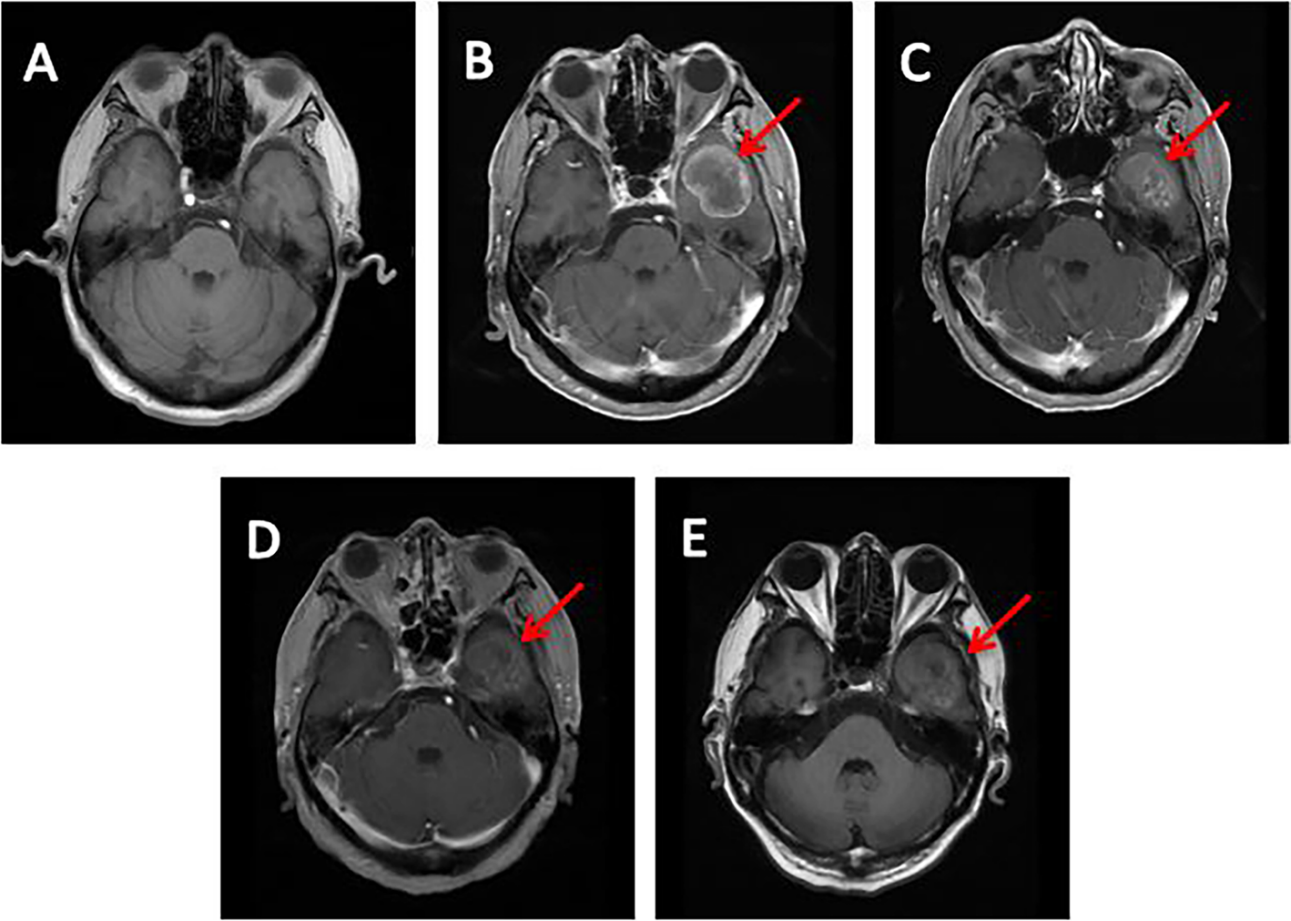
Brain magnetic resonance imaging (MRI) after treatment with afatinib and anlotinib. A Before any treatment. B Before afatinib treatment. C Brain MRI showing partial response after 1 month of afatinib and anlotinib. D Brain MRI showing stable disease after 5 months of afatinib and anlotinib. E Brain MRI showing progressive disease after 10 months of afatinib and anlotinib
